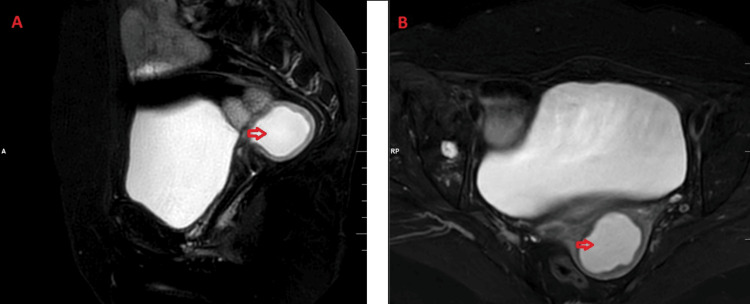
MRI T2-FS images of the pelvis (sagittal section: A, axial section: B) showing homogeneously hyperintense collection within the uterus. MRI: magnetic resonance imaging; FS: fat saturated
